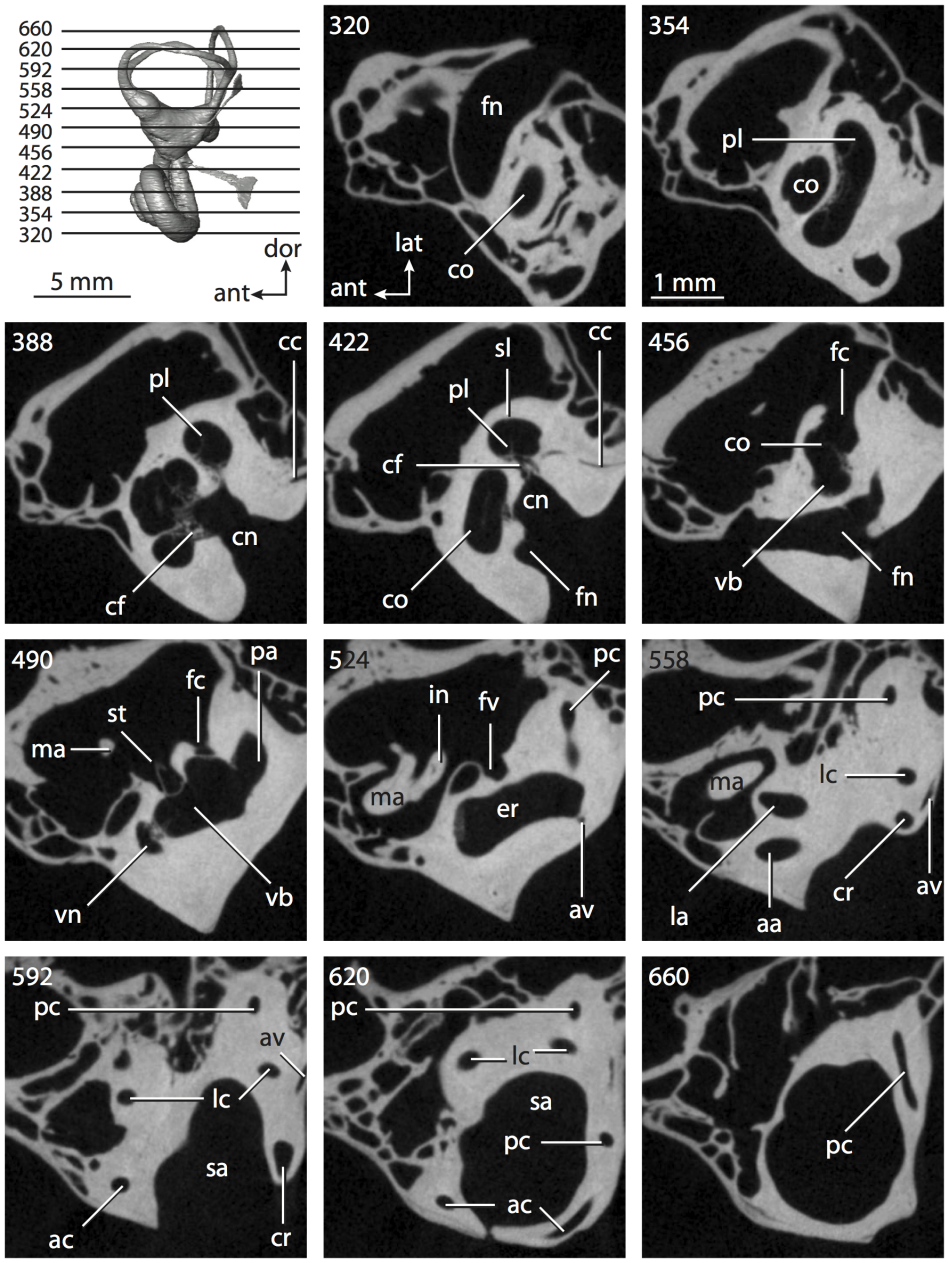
CT slices through ear region of*Macaca mulatta*. Abbreviations listed at the end of the Materials and Methods section.
